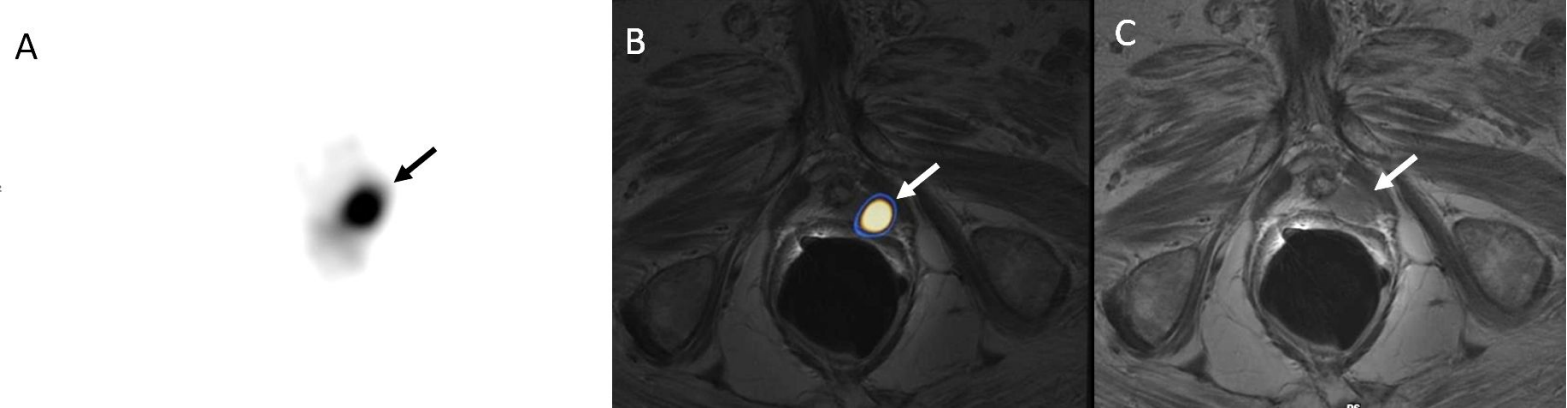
A 66-Year-Old Male with Newly Diagnosed Prostate Cancer (Gleason Score, 7) Transaxial PET slice (A) shows focal PSMA activity. Fused images (B) of ^68^Ga PSMA PET and (separately performed) MRI (C) show the PSMA activity localized to an apical solitary lesion at the left lobe of the prostate gland (arrows).
